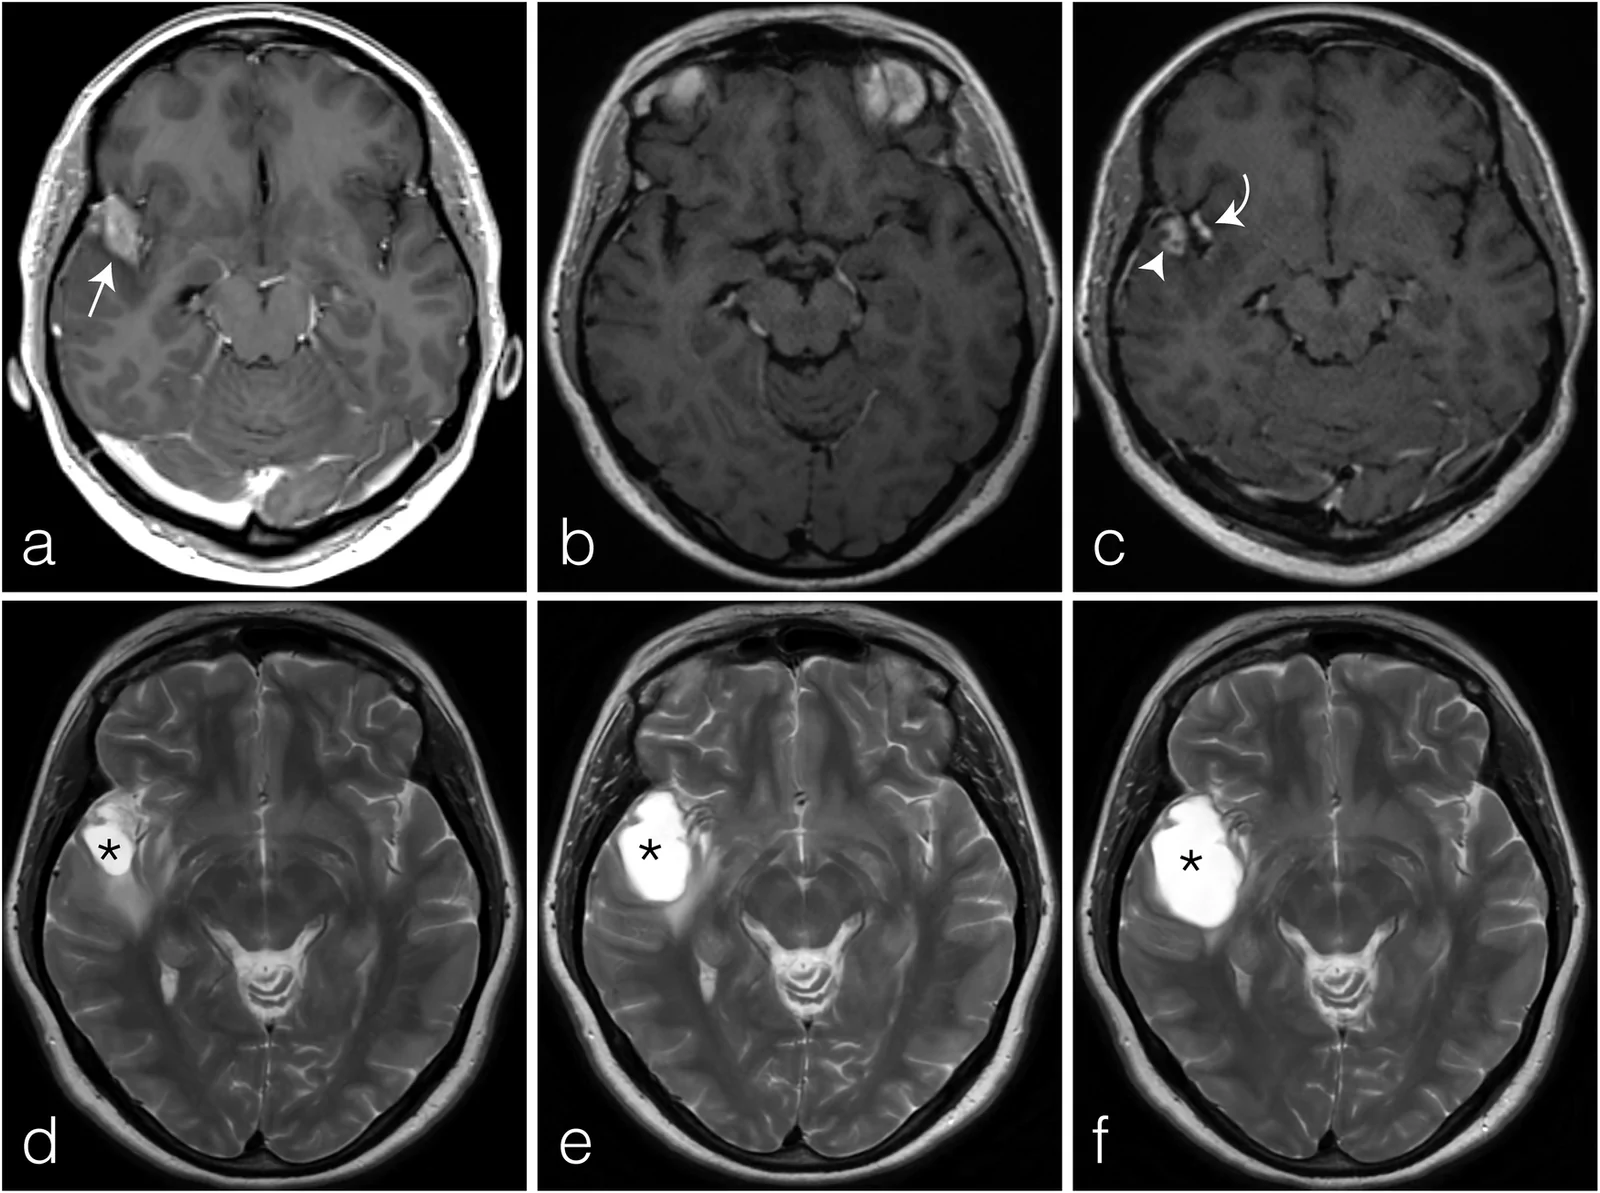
Cystic formation following stereotactic radiosurgery (SRS) of a brain metastasis. Serial MRI in a patient with breast cancer brain metastasis treated with SRS. a Axial post-contrast T1-weighted image at presentation: enhancing metastatic lesion in the right anterior temporal lobe (arrow). b Four months after SRS, axial post-contrast T1-weighted image: post-treatment response, with no appreciable residual lesion or enhancement at the treated site. c Two years after SRS, axial post-contrast T1-weighted image: small focus of enhancement at the original site (arrowhead) and a second focus on the opposing cortical bank across the adjacent sulcus (curved arrow). d–f Axial T2-weighted images obtained 3 years, 3 years 6 months, and 4 years after SRS, respectively: resolution of the enhancement and progressive cystic change at the treated site (asterisk), enlarging over time without solid or enhancing components
